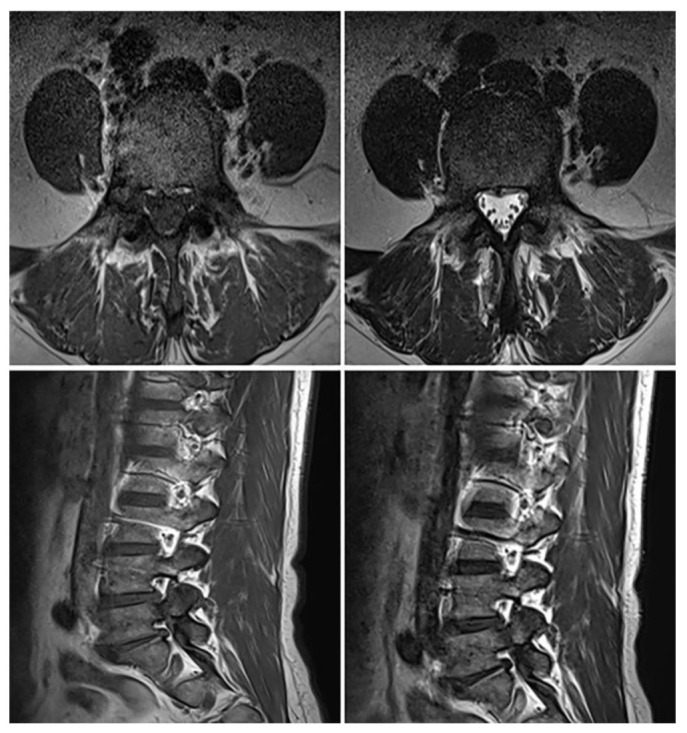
MRI showing low signal intensity on T1- and T2-weighted images in the bilateral L5 pedicles with minimal hyperintense marrow edema on T2, consistent with acute fractures. Additional findings include central disc protrusion at L3–4 and L4–5, and disc bulging with Modic type 2 change at L5–S1.
